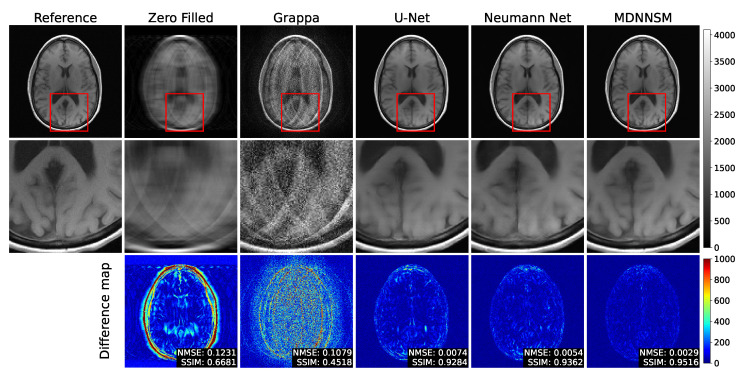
The top row shows fully sampled and reconstructed T1-weighted images using the zero-filled, Grappa, U-net, Neumann network, and MDNNSM methods, with an acceleration factor of 8 and an ACS rate 4%. The middle row shows detailed images zooming the red box area of the top row. The bottom row shows the difference between the reference and reconstruction images. The MDNNSM showed the lowest NMSE and the highest SSIM.
